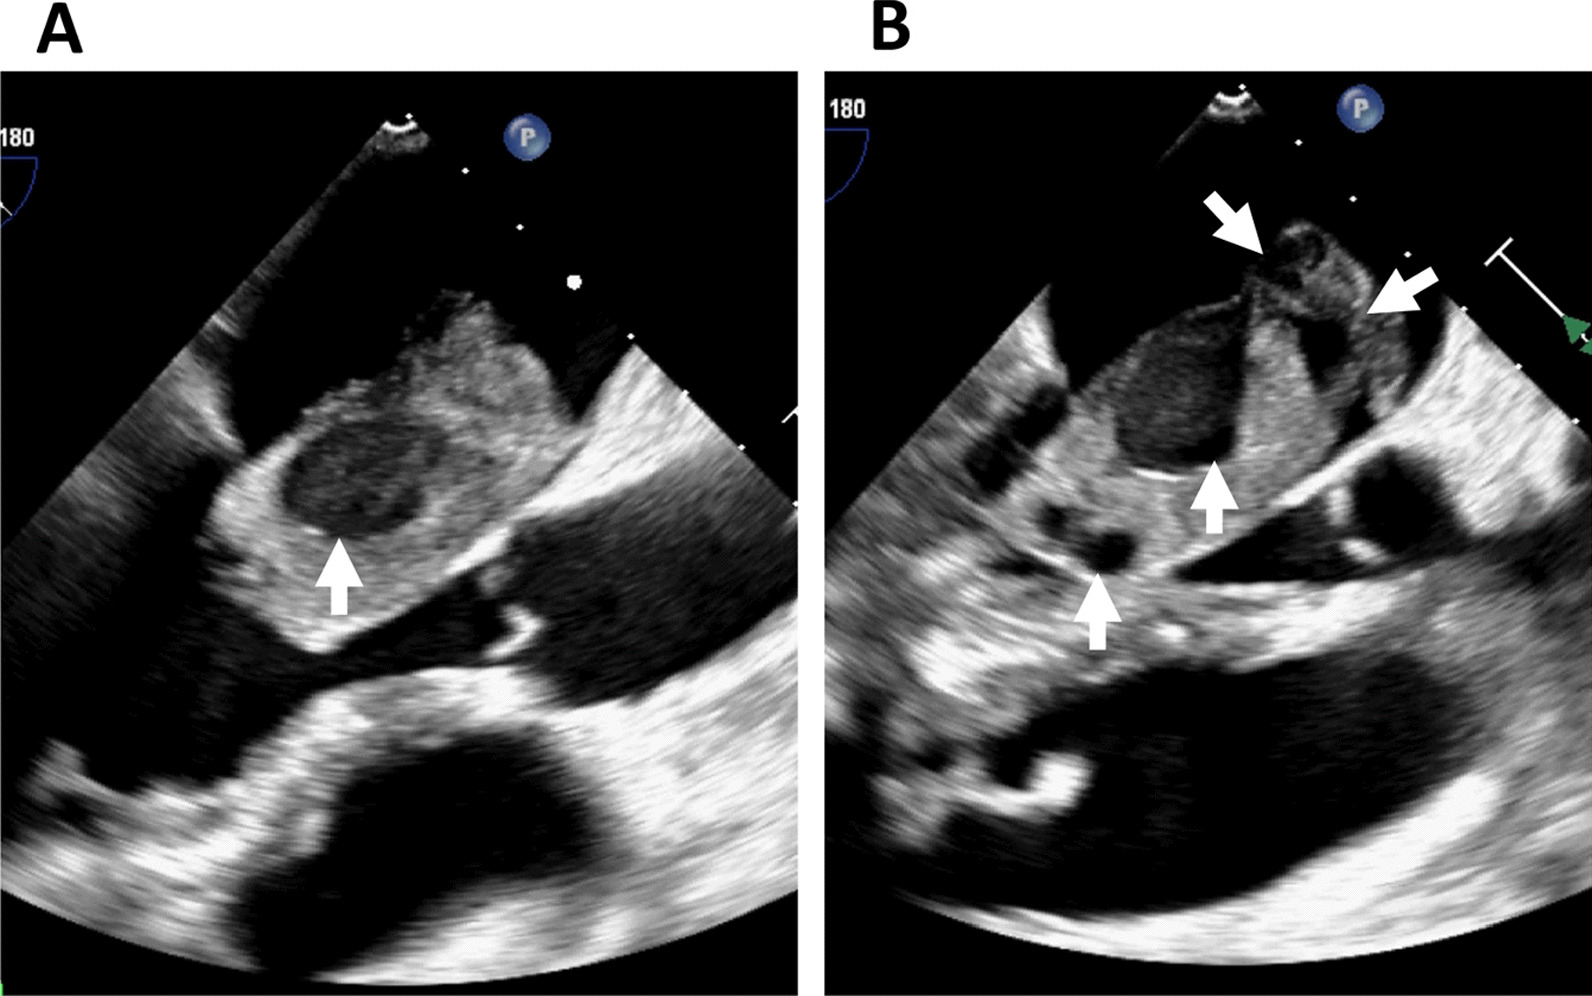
Transesophageal echocardiography images at 127 degrees. A At the insertion of the probe. B Just before establishing cardiopulmonary bypass. The tumor deformed rapidly morphologically during the operation. Flattening and lobing changes were remarkable. Small cystic formations just below the lobes were increased (arrows)
